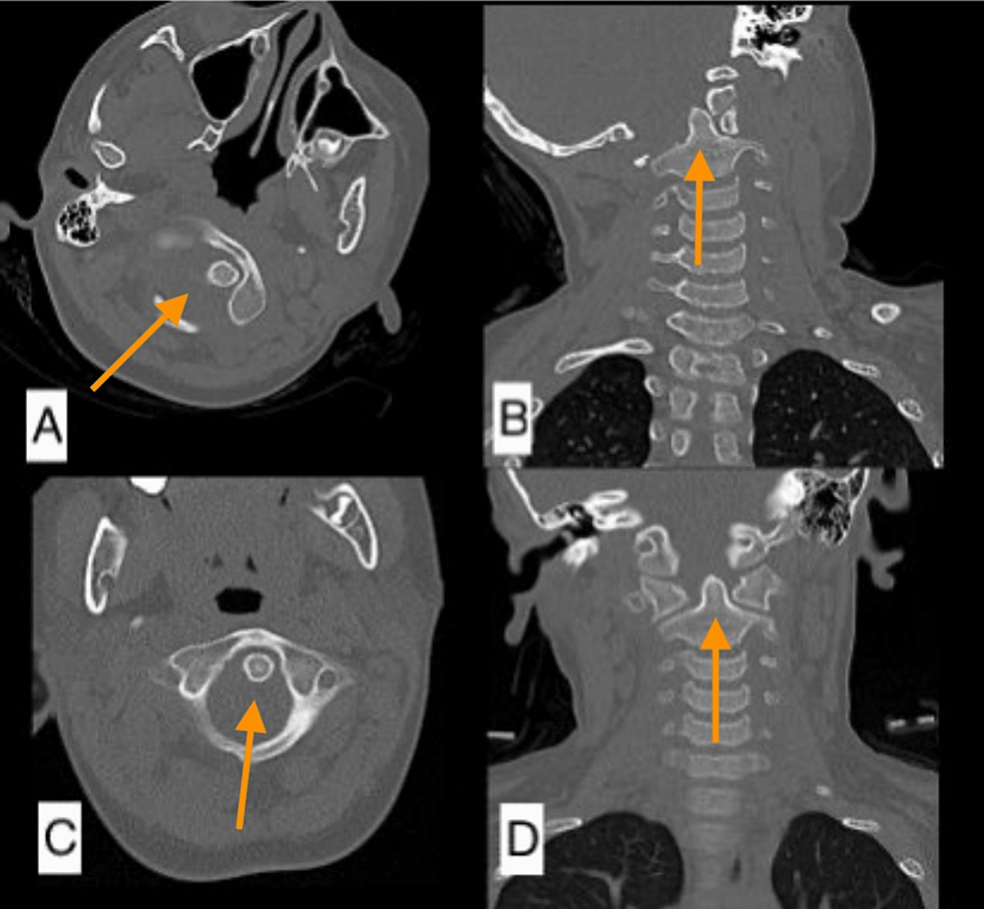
Pre-reduction CT cervical (A) axial view showing eccentrically positioned dens and (B) coronal view showing subluxation and rotation of the atlantoaxial joint complex (arrows) and post-reduction CT cervical (C) axial and (D) coronal view showing resolution of subluxation and restoration of alignment (arrows). CT: computed tomography
